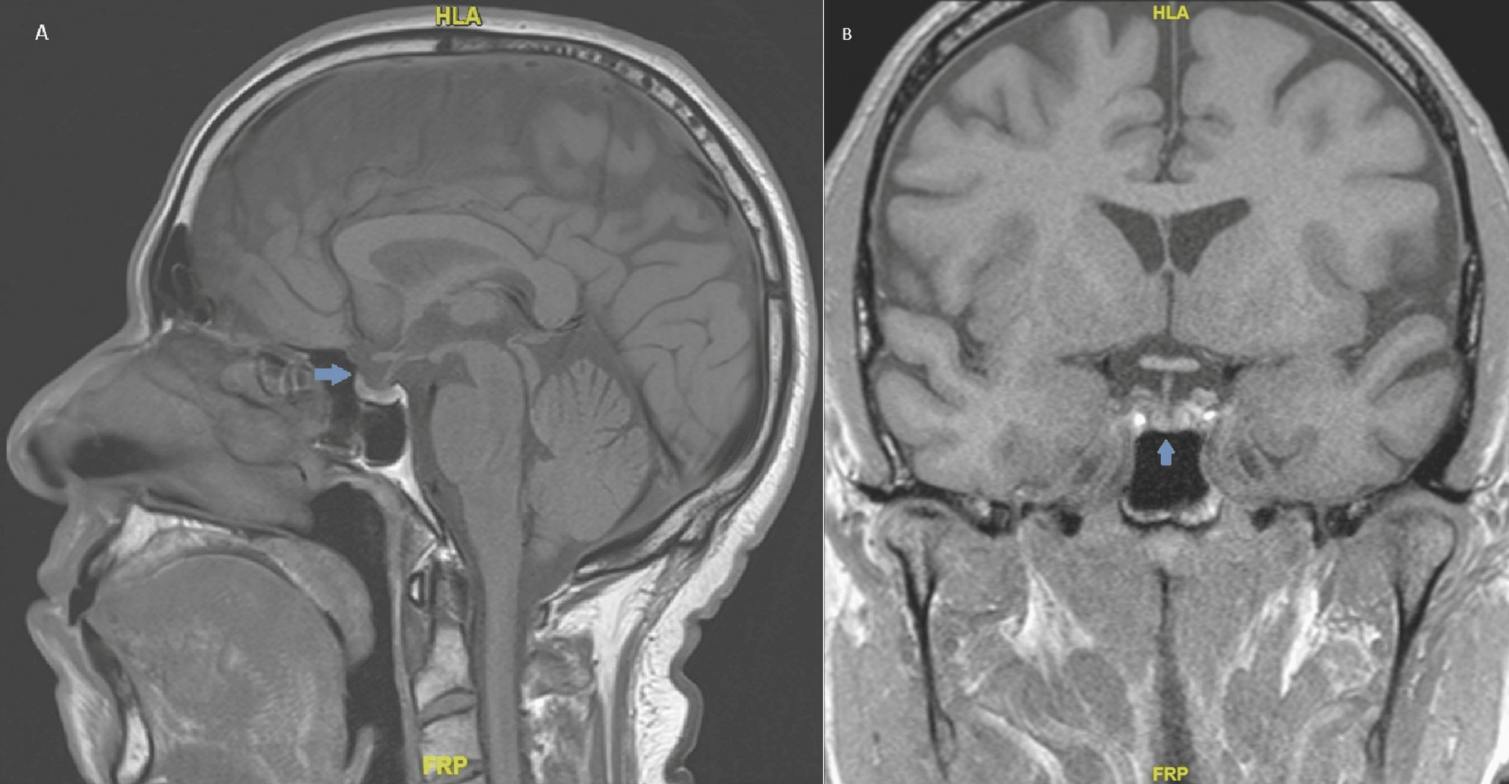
The arrow indicates a normal pituitary MRI in sagittal (A) and coronal (B) sections; no obvious pituitary gland mass.
